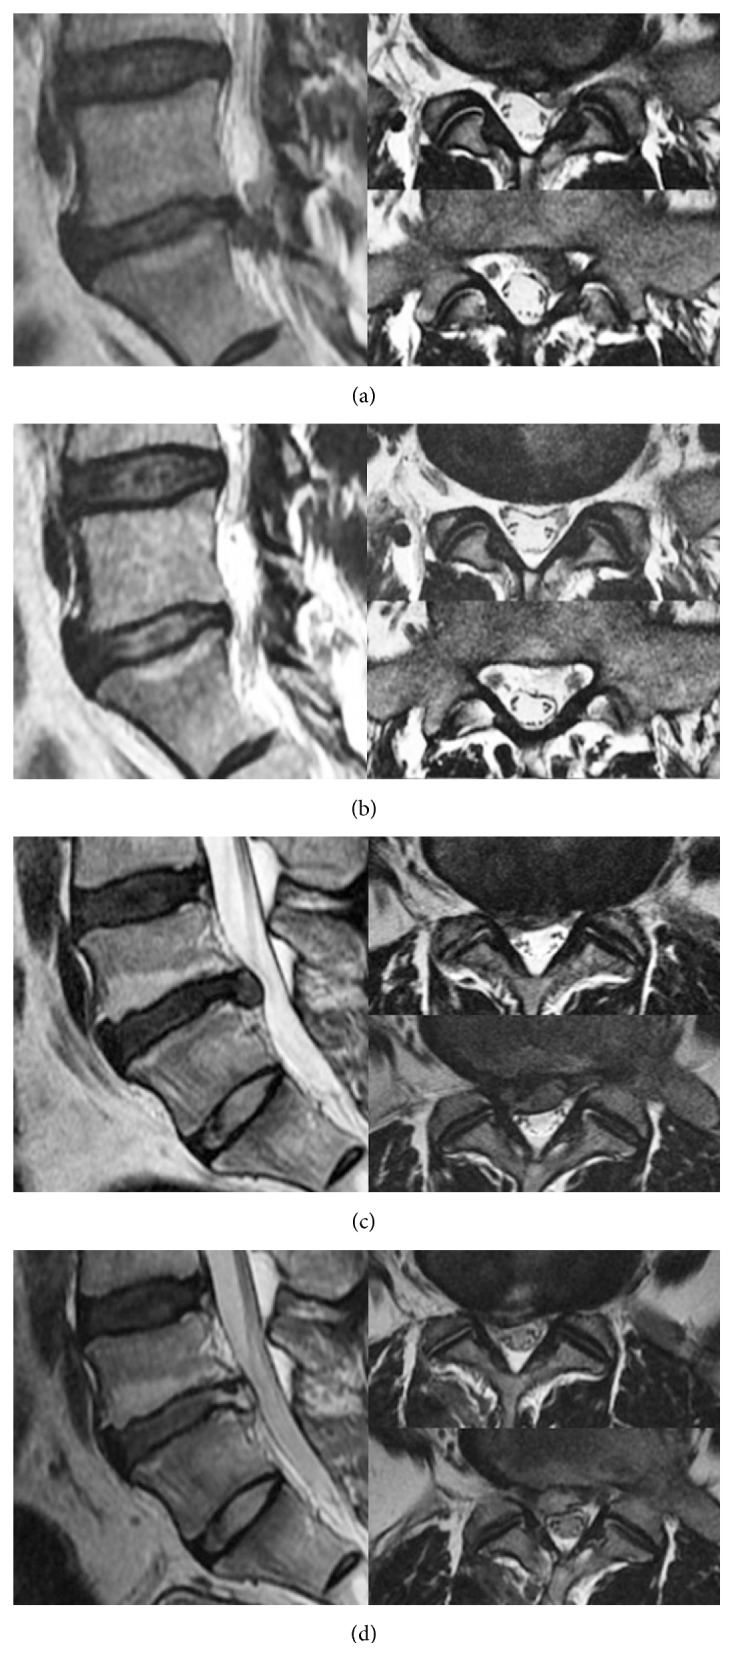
Radiological results using MRI. (a) and (b) Categorized as complete. Pre- and postoperative MRI showing the preserved ligamentum flavum, complete pathologic disc removal, and complete annulus restoration. (c) and (d) Categorized as sufficient. Pre- and postoperative MRI showing the preserved ligamentum flavum, complete pathologic disc removal, and incomplete annulus restoration.
